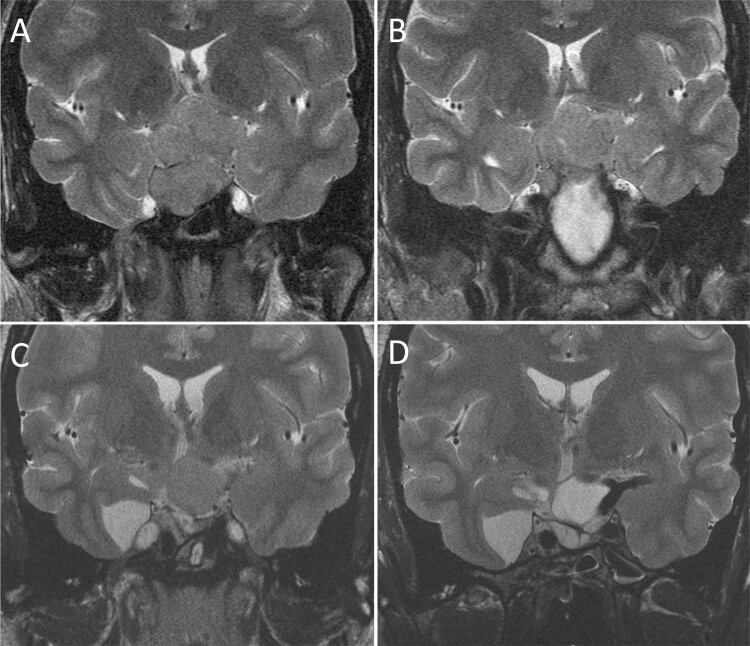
T2-weighted MRI scans at diagnosis, after first surgery, and before and after initiation of pasireotide LAR. T2-weighted MRI scan shows at diagnosis a large sellar mass with impingement of the optic chiasm and floor of the third ventricle and invasion into cavernous sinuses, right more than left (A). Postoperatively, T2-weighted MRI scan shows a large suprasellar tumor remnant (B). T2-weighted MRI scans showing tumor volume reduction from 8086 mm^3^ (C) before initiation of pasireotide to 5896 mm^3^ (D) during 15 months of treatment and increased T2-signal intensity after initiation of pasireotide LAR treatment: ROI adenoma/normal pituitary ratio before pasireotide LAR treatment was 1.2 (C) and increased to 2.0 (D). Abbreviations: LAR, long-acting release; MRI, magnetic resonance imaging; ROI, region of interest.
